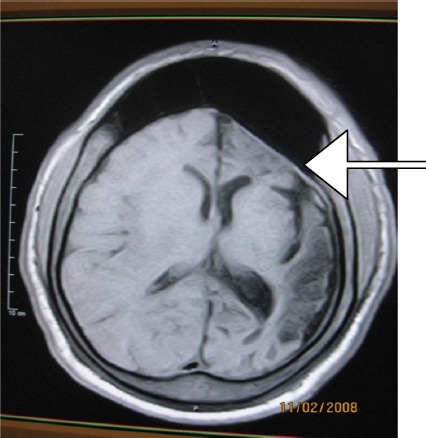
T1W axial MR image showing left hemicerebral atrophy with hypointense parietal lesion, enlargements of frontal sinus and left atrium
